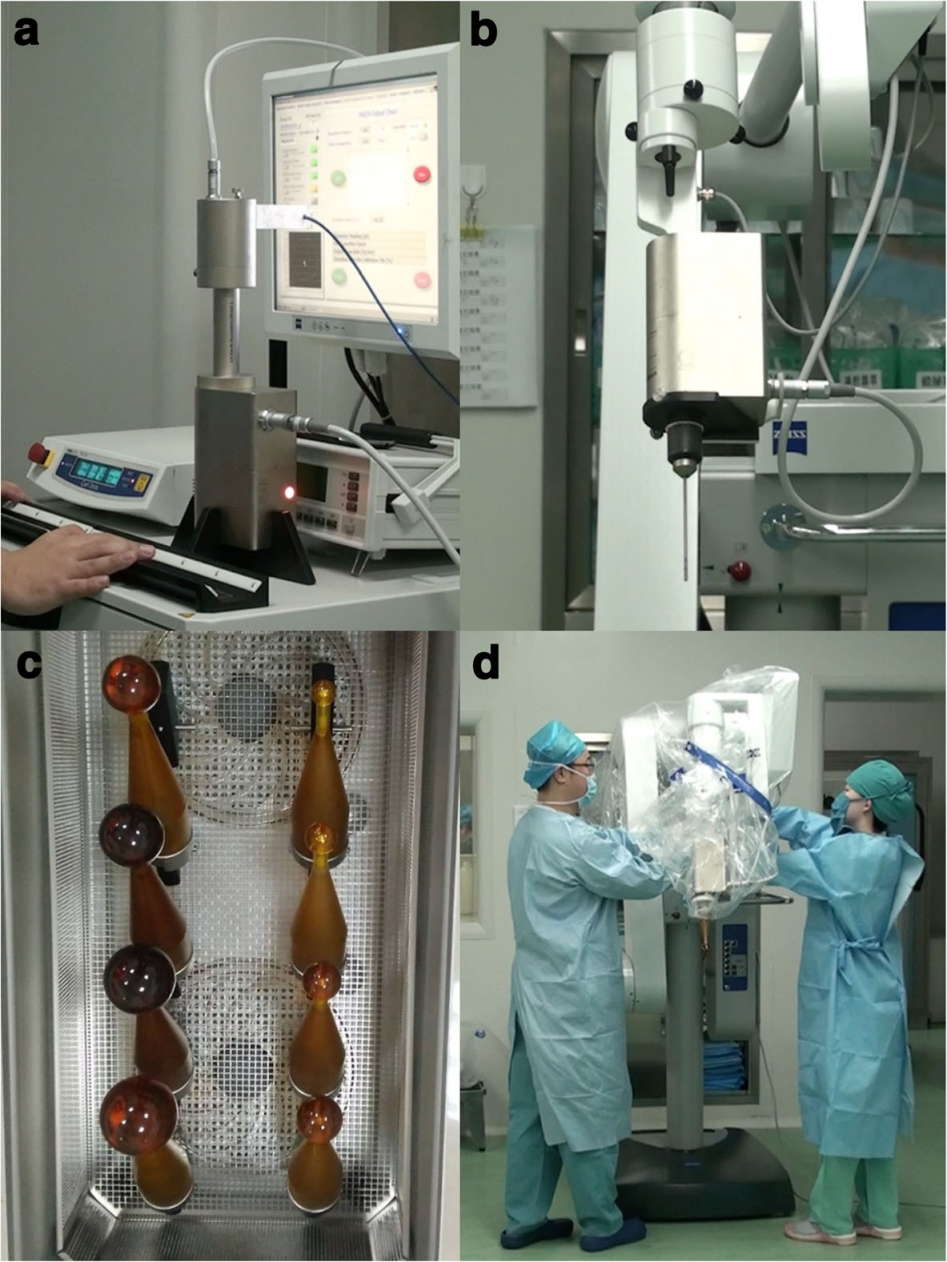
The introduction of Intrabeam System. The Zeiss Intrabeam System (Carl Zeiss Meditec AG, Germany). a The console. b XRS. c Spherical plastic applicators for IORT. d The X-rays source (XRS) with a spherical applicator mounted on the floor stand
